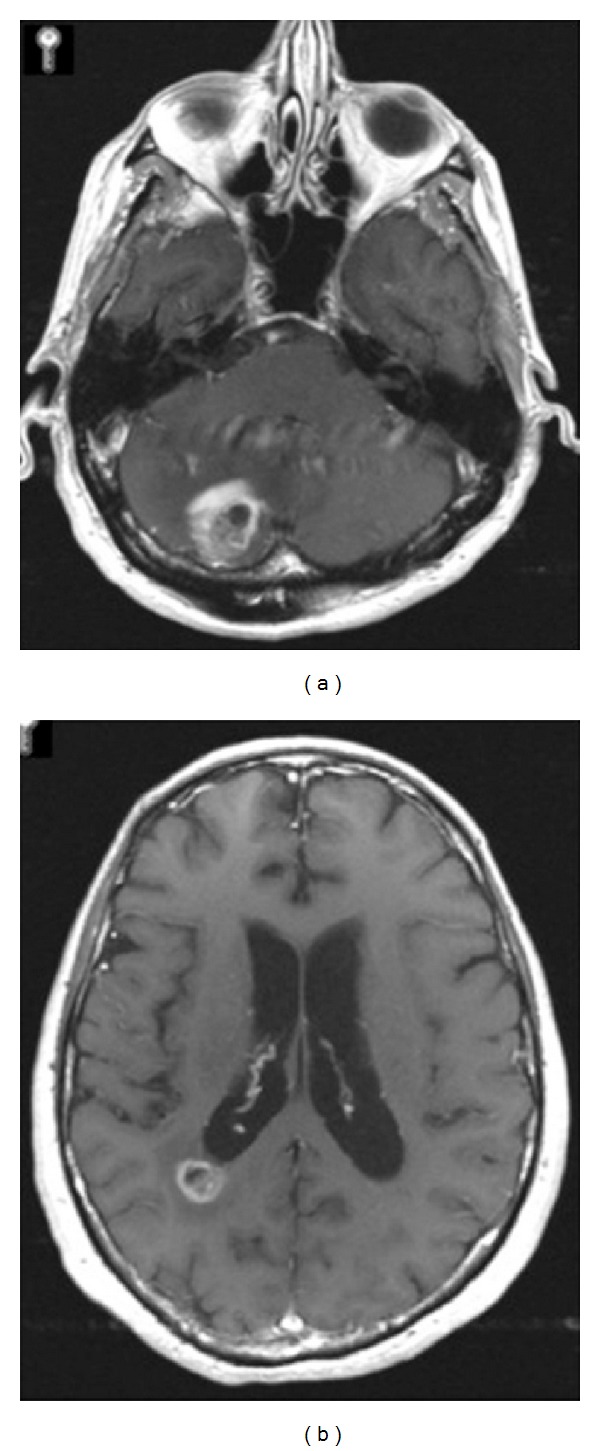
Preoperative MR imaging. (a) Axial T1-weighted image with contrast at the level of the cerebellum showing an enhancing mass in the right cerebellar hemisphere measuring 2.9 cm × 2.4 cm. (b) Axial T1-weighted image with contrast at the level of the lateral ventricles demonstrating an enhancing lesion in the right posterior periventricular region measuring 1.0 cm × 1.4 cm.
